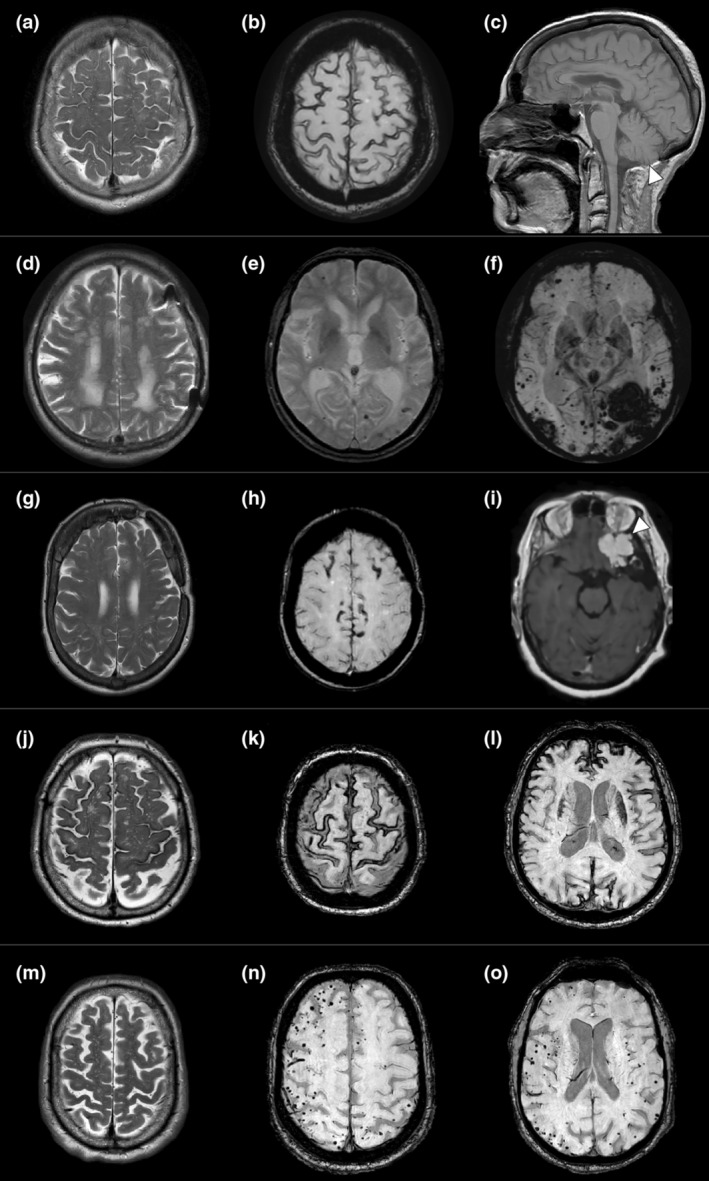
Each row shows representative MRI images for the five patients. The left column shows T2‐weighted imaging. The middle and right column show blood sensitive imaging (either susceptibility‐weighted imaging or gradient‐echo T2‐weighted imaging) unless otherwise stated. Case 1: enlarged perivascular spaces in the centrum semiovale (CSO‐EPVS) (a), cortical superficial siderosis (cSS) (b), and the foramen magnum decompression for a Chiari I malformation ((c) white arrowhead, sagittal T1‐weighted image). Case 2: severe burden of small vessel disease (d) and progressive cerebral microbleeds and the development of an intracerebral haemorrhage (e, f). Case 3: enlarged perivascular spaces (g), convexity cSS (h) and residual sphenoid meningioma ((i) white arrowhead, gadolinium‐enhanced T1‐weighted image). Case 4: enlarged perivascular spaces and multispot pattern of small vessel disease (j) and extensive superficial siderosis (k, l). Case 5: enlarged perivascular spaces (m) and innumerable cerebral microbleeds but no cSS (n, o).
